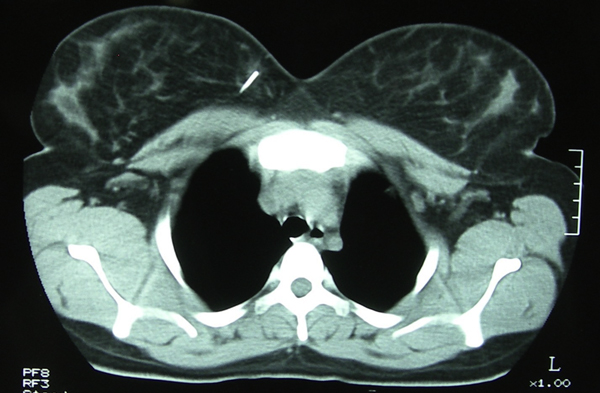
Computed tomography of the thorax revealing the needle approximately 1.5 cm in length in the right breast.
